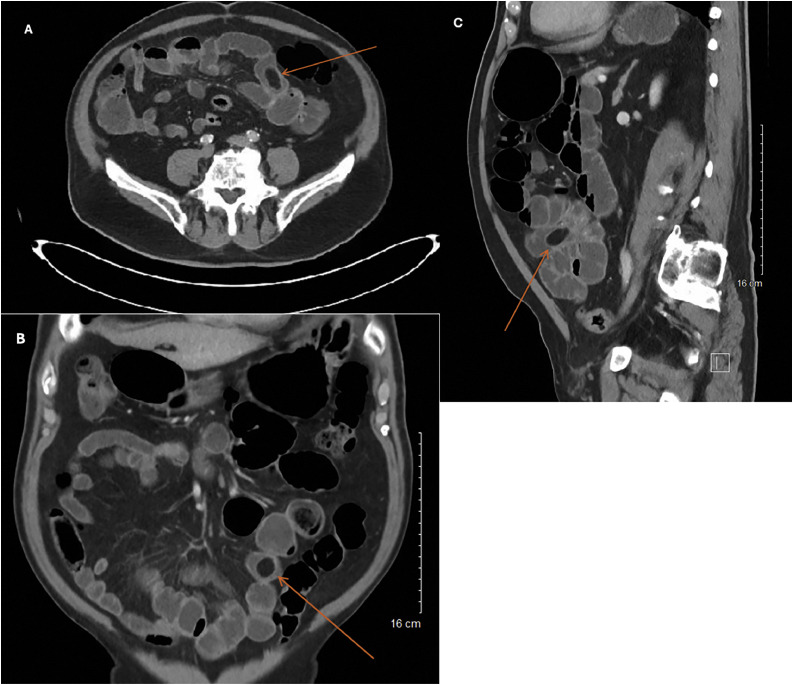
Axial (A), coronal (B), and sagittal (C) abdominal CT images on readmission, significant for filling defect consistent with an obstructive agent (orange arrow).
